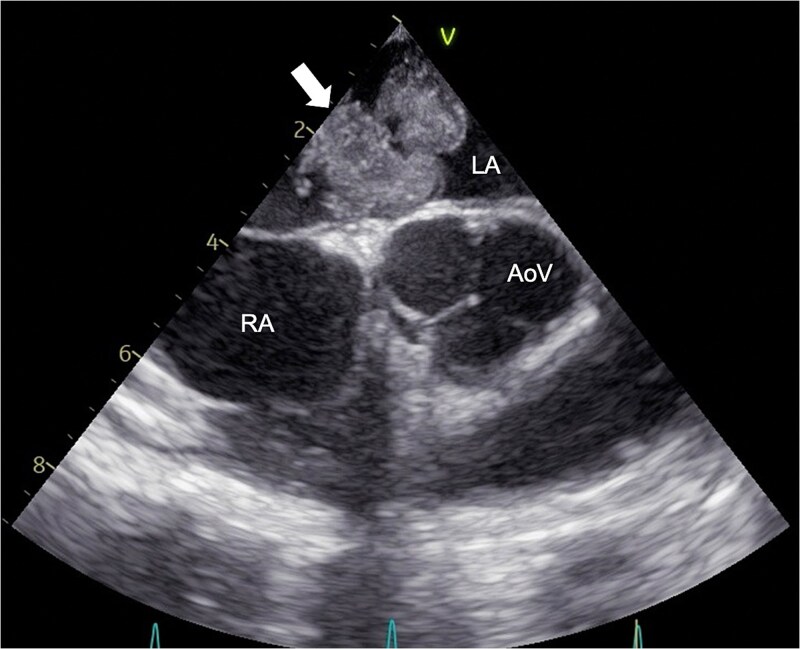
Preoperative echocardiography. Transesophageal echocardiography showing a 40-mm pedunculated tumor (arrowed) attached to the LA side of the atrial septum. RA, right atrium; LA, left atrium; AoV, aortic valve.
